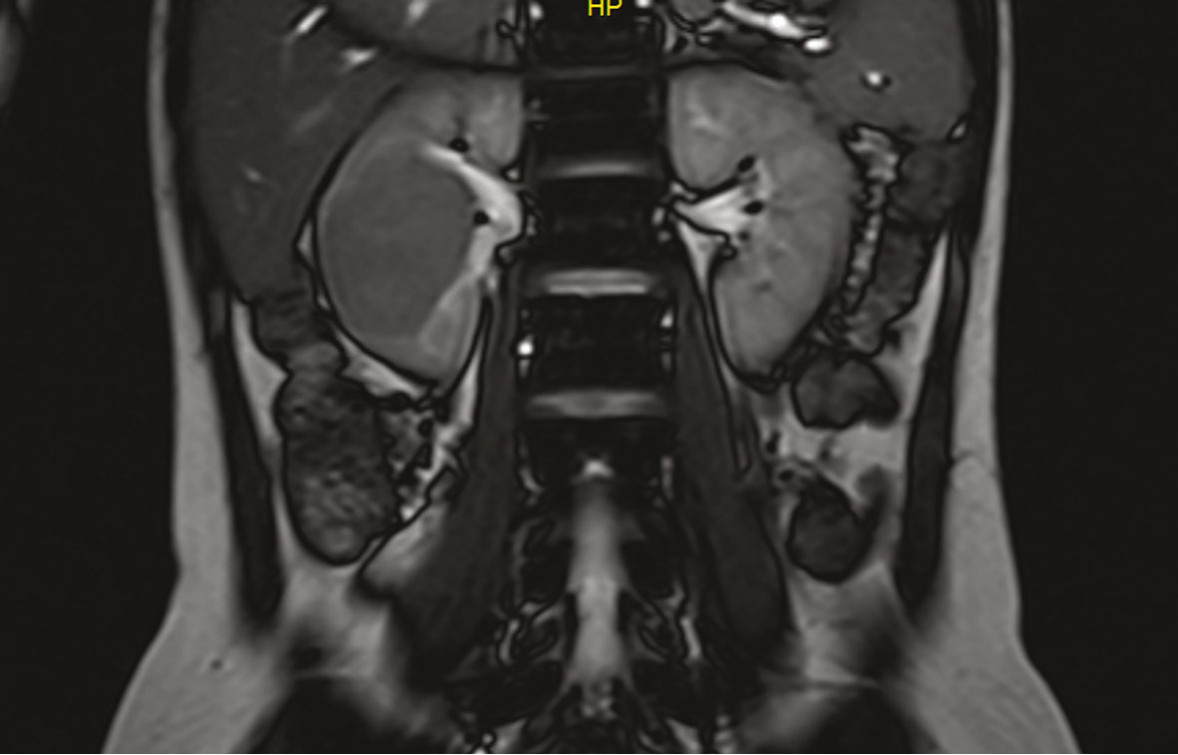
T2-weighted coronal section of the renal mass. Uterus containing fetus was visible in more anteriorly sections.
